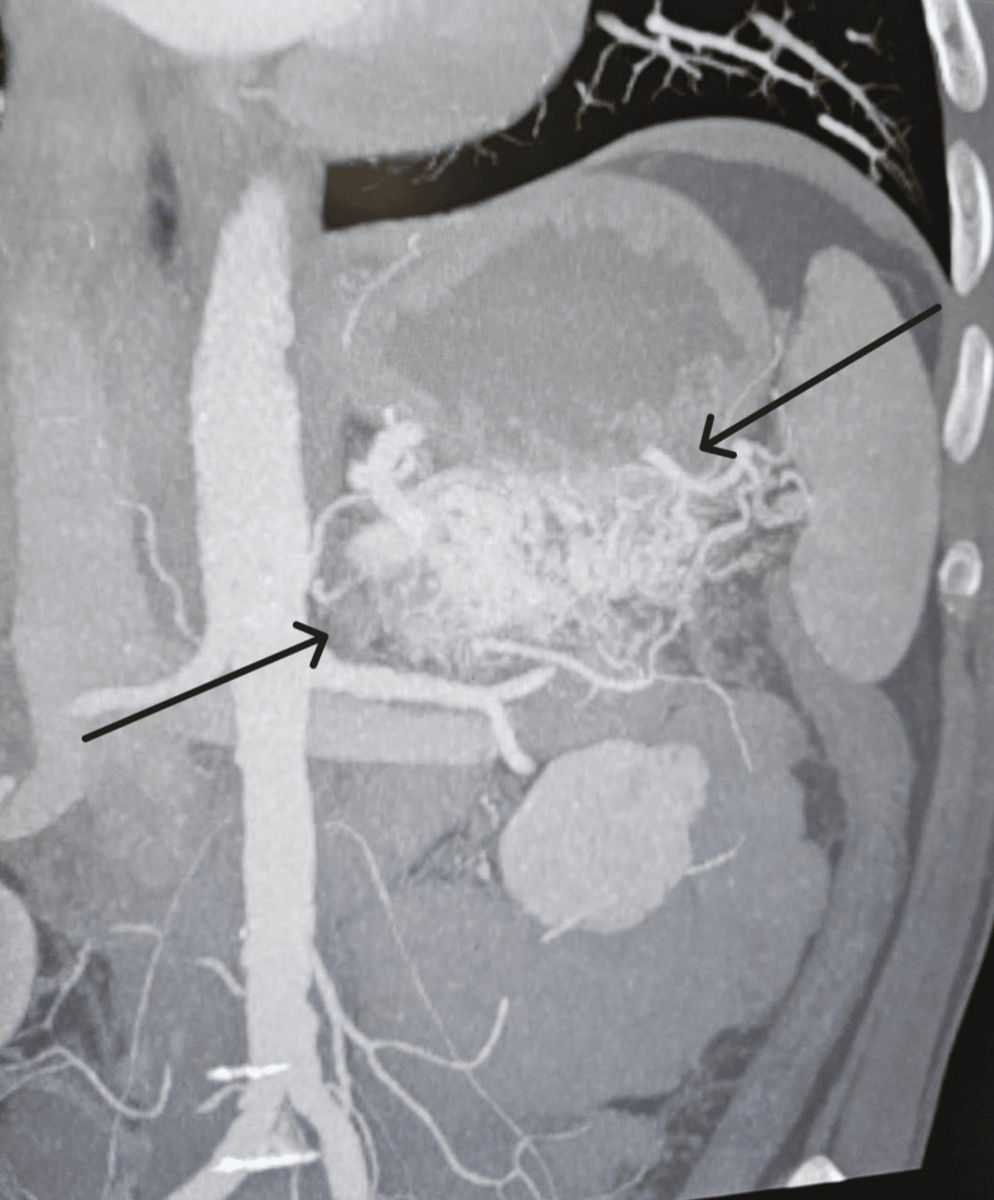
A CT scan of the abdomen and pelvis with contrast showing a pancreatic tail arteriovenous malformation.
